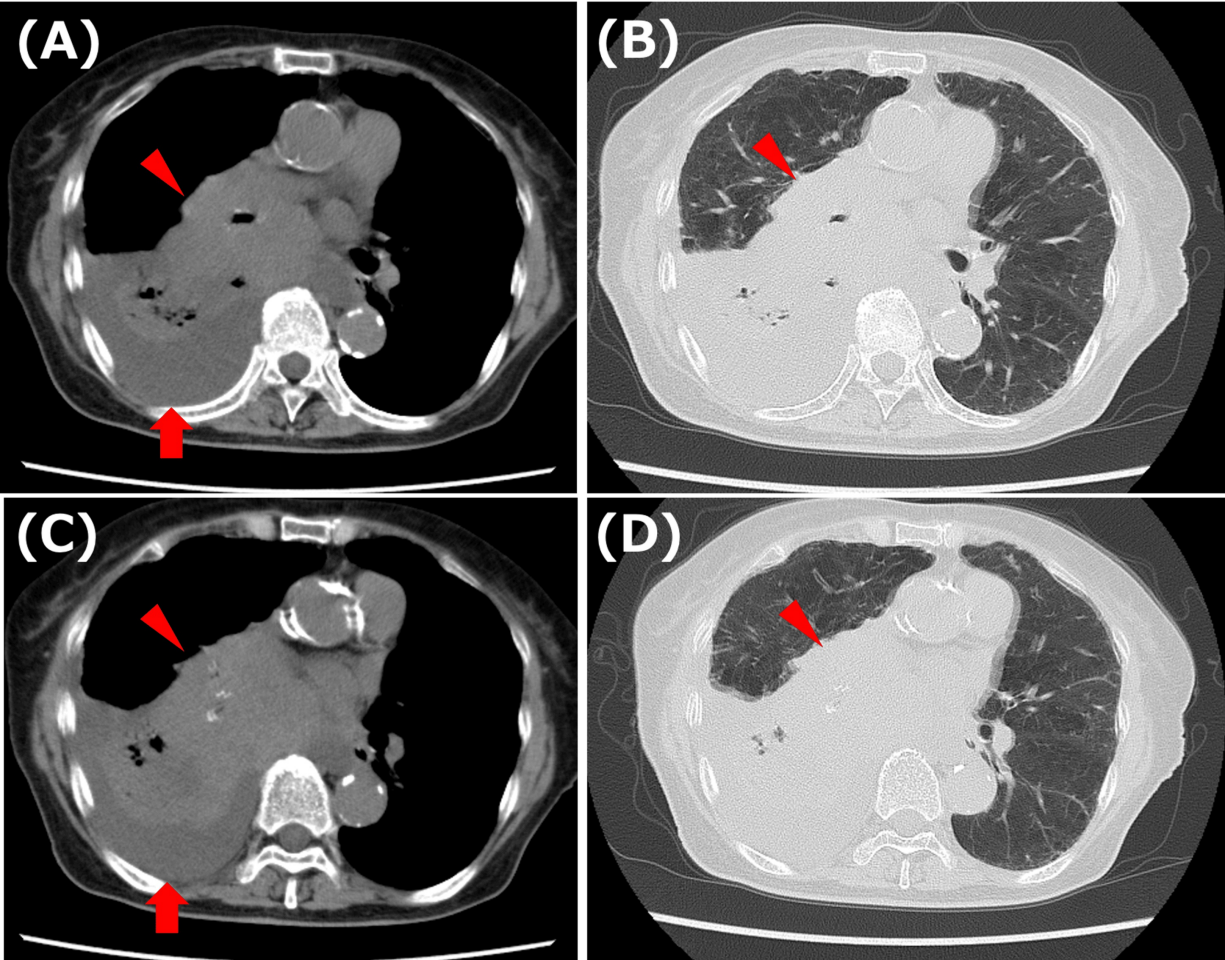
Chest CT findings on admission. Axial CT images in the mediastinal window (A, C) and corresponding lung-window (B, D) images demonstrate a right hilar mass (arrowheads) with a right pleural effusion (arrows).
